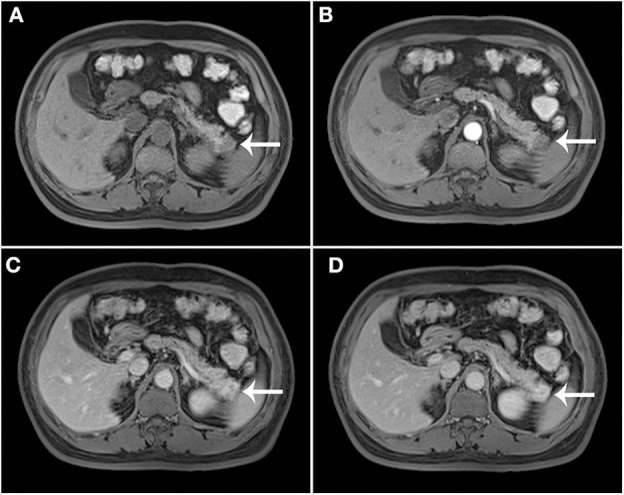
Post-contrast MRI. T1WI without contrast enhancement (A), the arterial phase (B), the portal (C), and the late phases (D).
